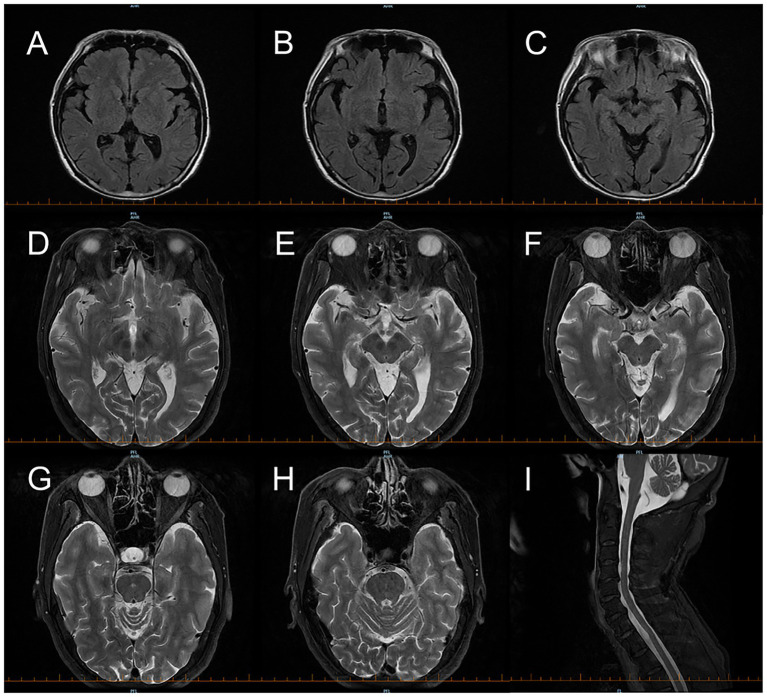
Brain MRI of the 62-year-old male patient demonstrates no typical features of Wernicke encephalopathy. (A–C) Axial FLAIR sequence: No abnormal hyperintensities are seen in the mammillary bodies, thalamus, periaqueductal gray matter, or brainstem. (D–H) Follow-up thin-section axial T2-weighted skull base images show no definite abnormal signals. Cervical spinal cord MRI (I) (T2-weighted) shows no characteristic lesions of subacute combined degeneration.
